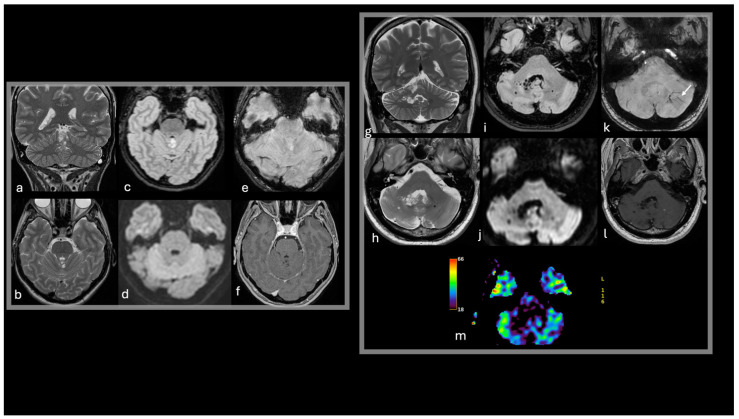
Imaging features suggestive of infratentorial MVNT. T2w coronal images (a,g), T2w axial images (b,h), FLAIR axial images (c,i), DWI (d,j), SWI (e,k), post-contrast T1w (f,l), DSC-CBV map (m).
